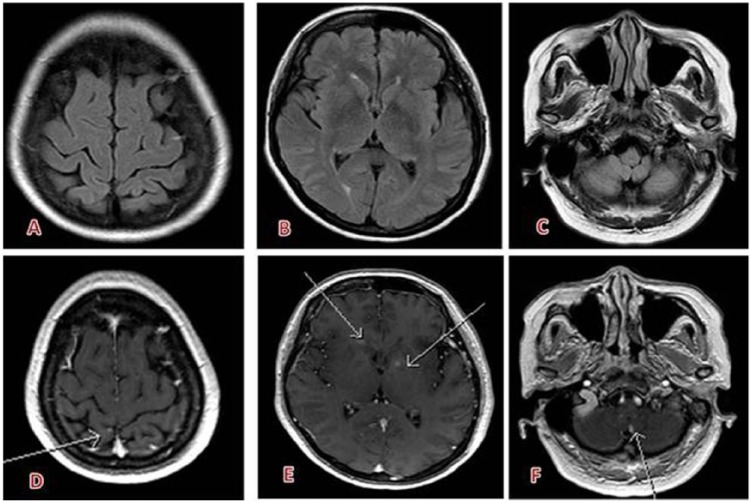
Multiple small brain metastases (breast cancer) visible only on T1-weighted sequences after intravenous contrast injection (gadobutrol, 7.5 mL) (D) right parietal lesion, (E) left lenticular and right head of caudate lesions, (F) left cerebellar lesion and not on (A-C) fluid-attenuated inversion recovery sequence.
